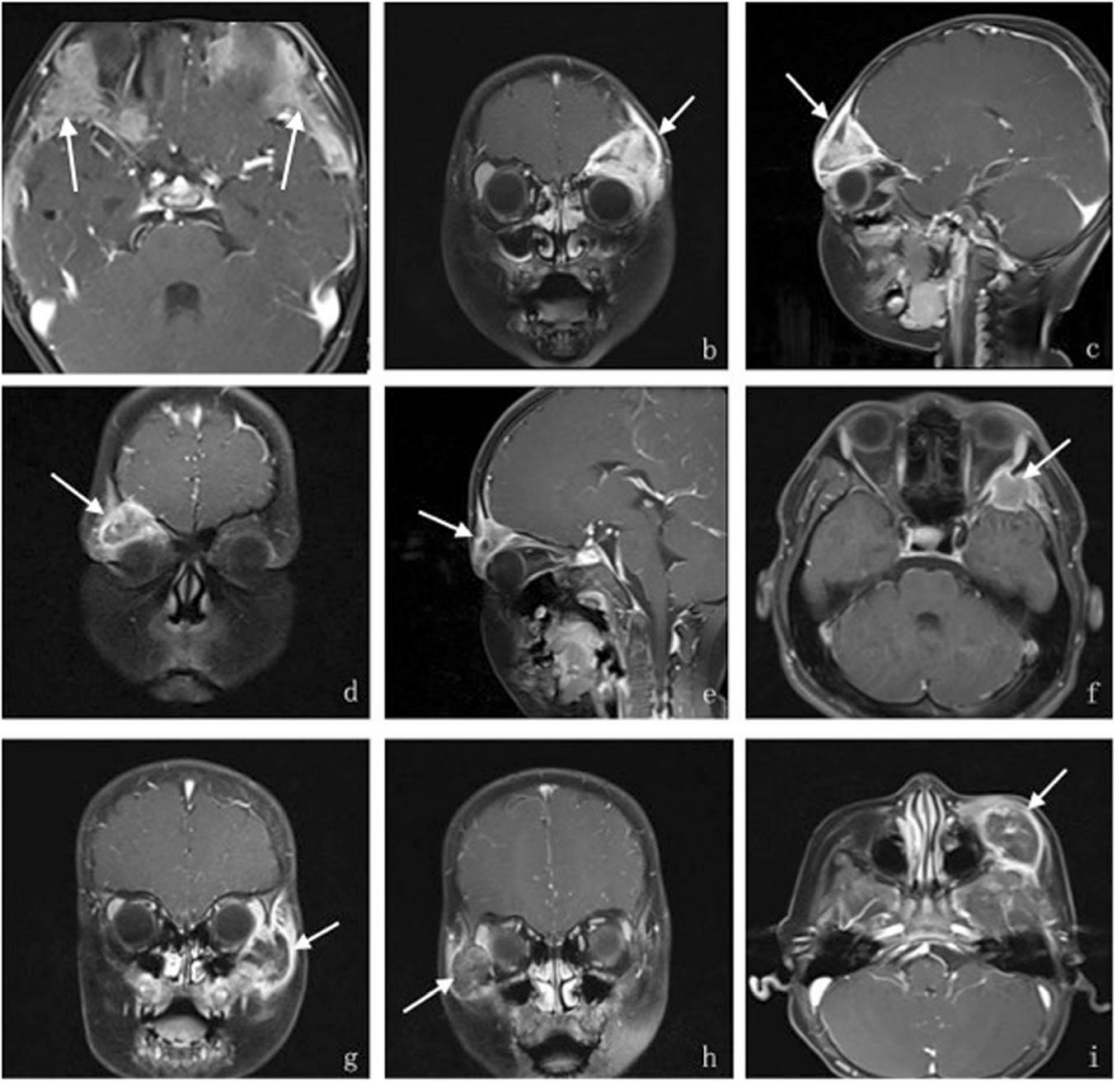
a-i. The different performances of orbital LCH lesions in enhanced T1-weighted images. Axial T1-weighted postcontrast images (a) demonstrate homogeneous enhancement; Coronal (b) and sagittal(c) T1-weighted postcontrast images represent the same patient with a lesion located in the superolateral of the left orbit, the lesion shaped like a triangle crumb presenting significant heterogeneous enhancement with some irregular low signal areas near the lesion edge. Coronal (d) and sagittal(e) T1-weighted postcontrast images represent the same patient, the lesion is located in the superolateral right orbit with an irregular triangle shape, an unclear boundary, and slight or moderate heterogeneous enhancement. Axial T1-weighted postcontrast images (f) demonstrate a lesion located in the lateral wall of left orbit and shows slight homogeneous enhancement around the center of the lesion. Axial (g) and Coronal (h) T1-weighted postcontrast images represent the same patient with a lesion showing flocculent enhancement in the central hypointense area, and the area surrounding the lesion showed more significant enhancement. Coronal (i) T1-weighted postcontrast images showing slight-moderate heterogeneous enhancement in the center of the lesion, and surrounded by a circular low signal
